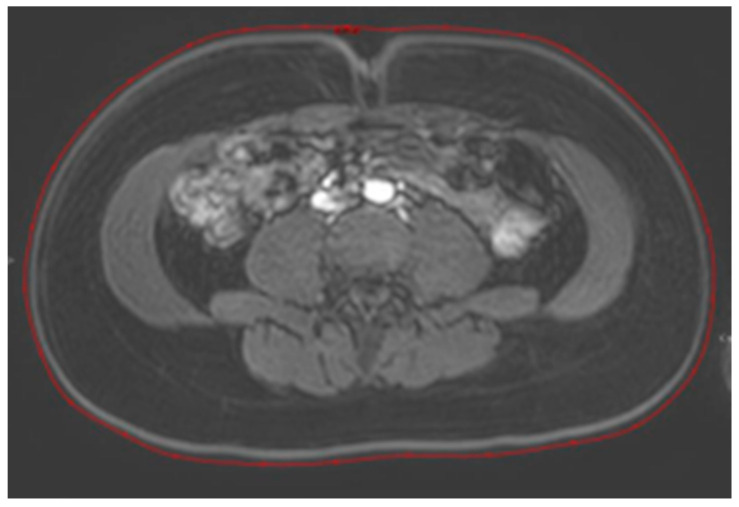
Measurement of the abdominal circumference on MRI. Measurements were made on axial images of the dynamic contrast series at the level of the umbilicus by surrounding the image of the skin (red line).
